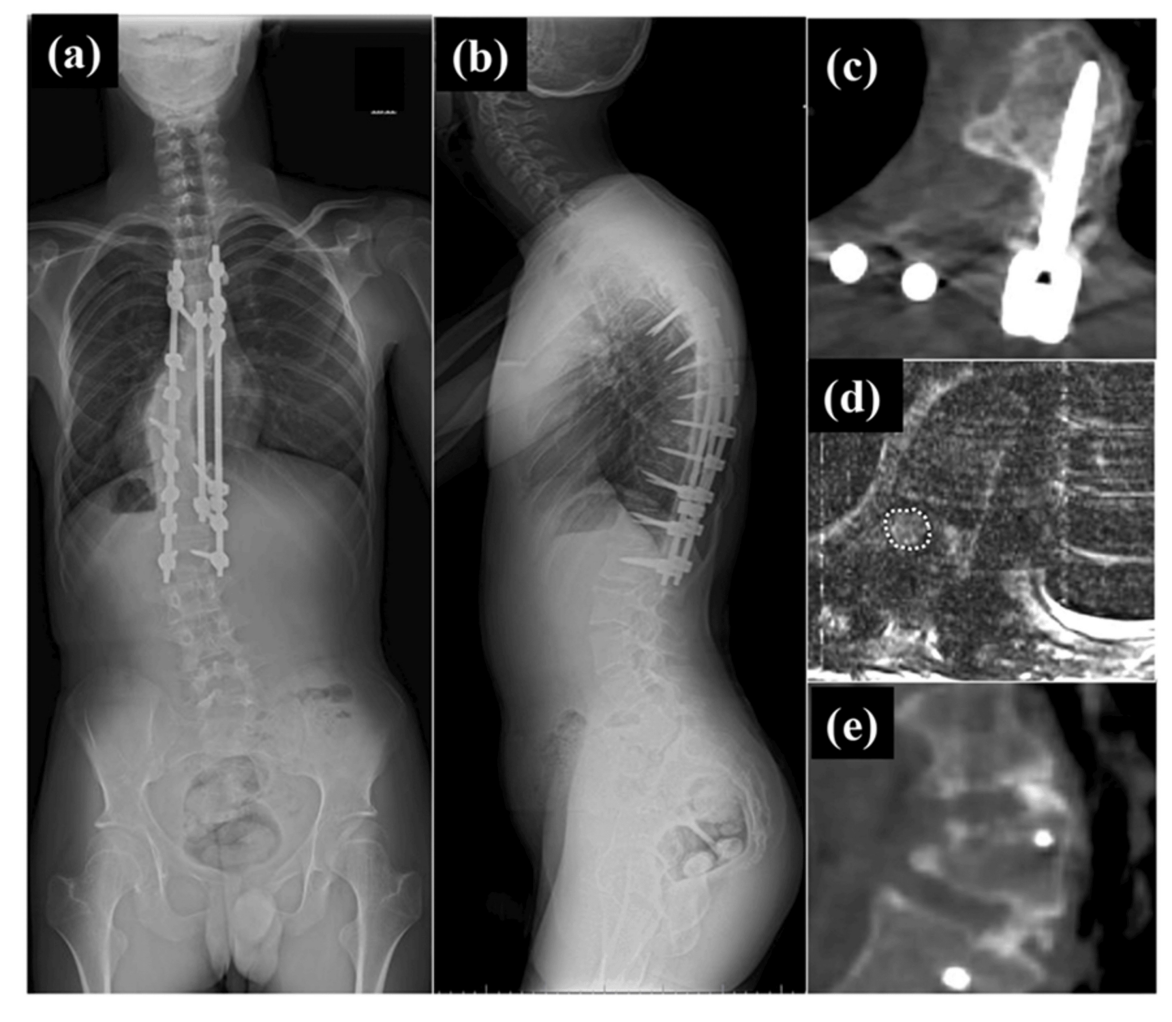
Postoperative coronal and sagittal standing full-spine radiograph, computed tomographic (CT) and magnetic resonance (MR) images At 1.5 years after surgery, coronal standing full-spine radiograph showed the Cobb angle of the scoliotic major curve from T8 to T11 was 40 degrees (a), and sagittal standing full spine radiograph showed local sharp kyphosis, whose Cobb angle was 45 degrees, became less outstanding and thoracic kyphosis (T5-12) was 50 degrees (b). Axial CT image at one year after surgery showed that the right T10 pedicle and the part of the posterior and lateral walls of the T10 vertebral body was resected. (c). Axial MR image at six months after surgery showed the spinal cord (area enclosed by white dashed line), which had been as flattened preoperatively, was depicted as an oval (d). Coronal CT image at one year after surgery showed bone union was obtained in T9/10 and 10/11 levels performed with anterior bone grafting.
